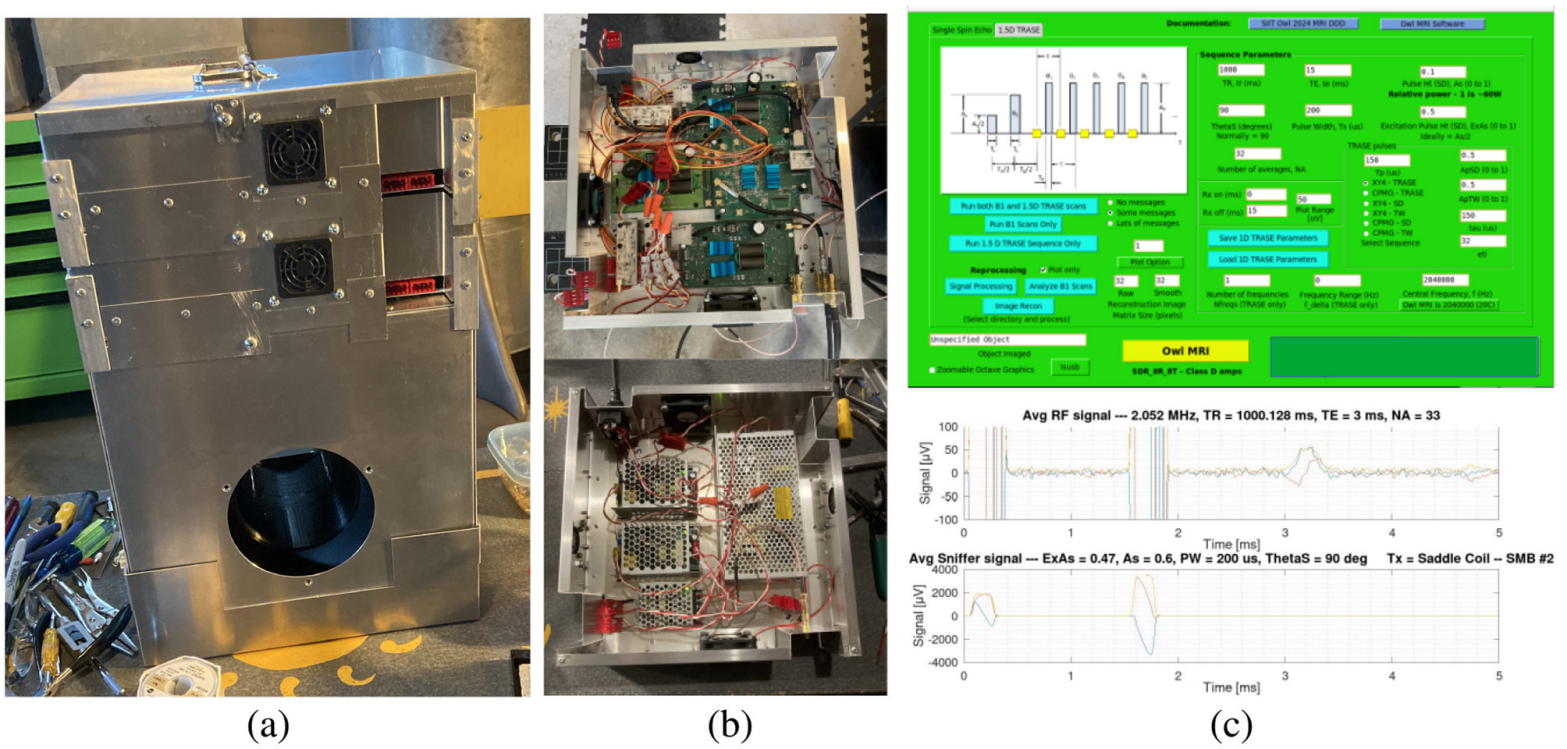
(A) The retrofitted *Owl MRI* of Sarty and Vidarsson (2018) referred to as the *USask Owl MRI* in the text. (B) The magnet is installed in the bottom box with power supplies mounted in a tray above the magnet (bottom image) and the electronics—class D RF amplifiers and an SDR (see text) are mounted in a top tray (and shown in the top image). (C) User interface for a TRASE sequence (top image) and an example spin echo output along with the measurement of the RF pulses via a permanently mounted sniffer loop. The *USask Owl MRI* has only produced low-resolution images to date. (A redesign of the thin saddle coil is in progress to resolve that).
